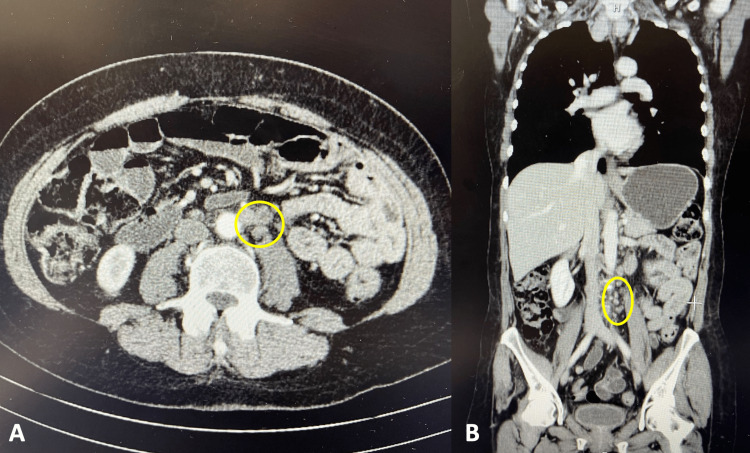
Patient's abdominal CT (A: axial plane; B: coronal plane) Abdominal CT shows several small lymph nodes in the intercavoaortic and lateroaortic regions (marked).
